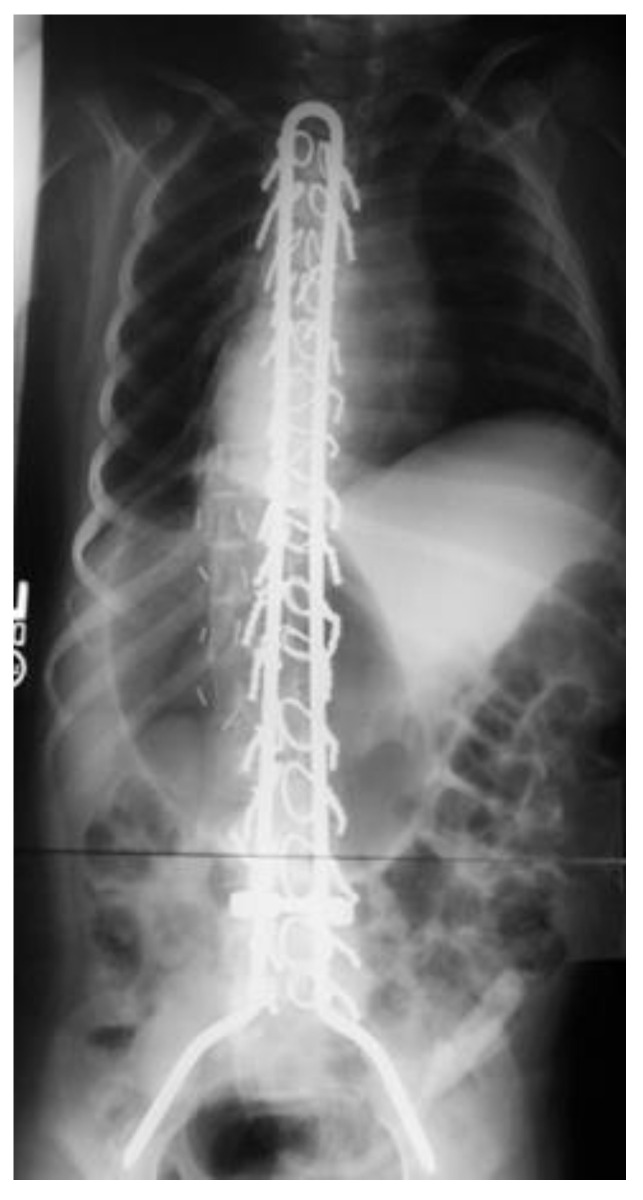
Anterior-posterior radiograph of a Unit rod construct using Luque wiring used for the treatment of a patient with neuromuscular scoliosis.
